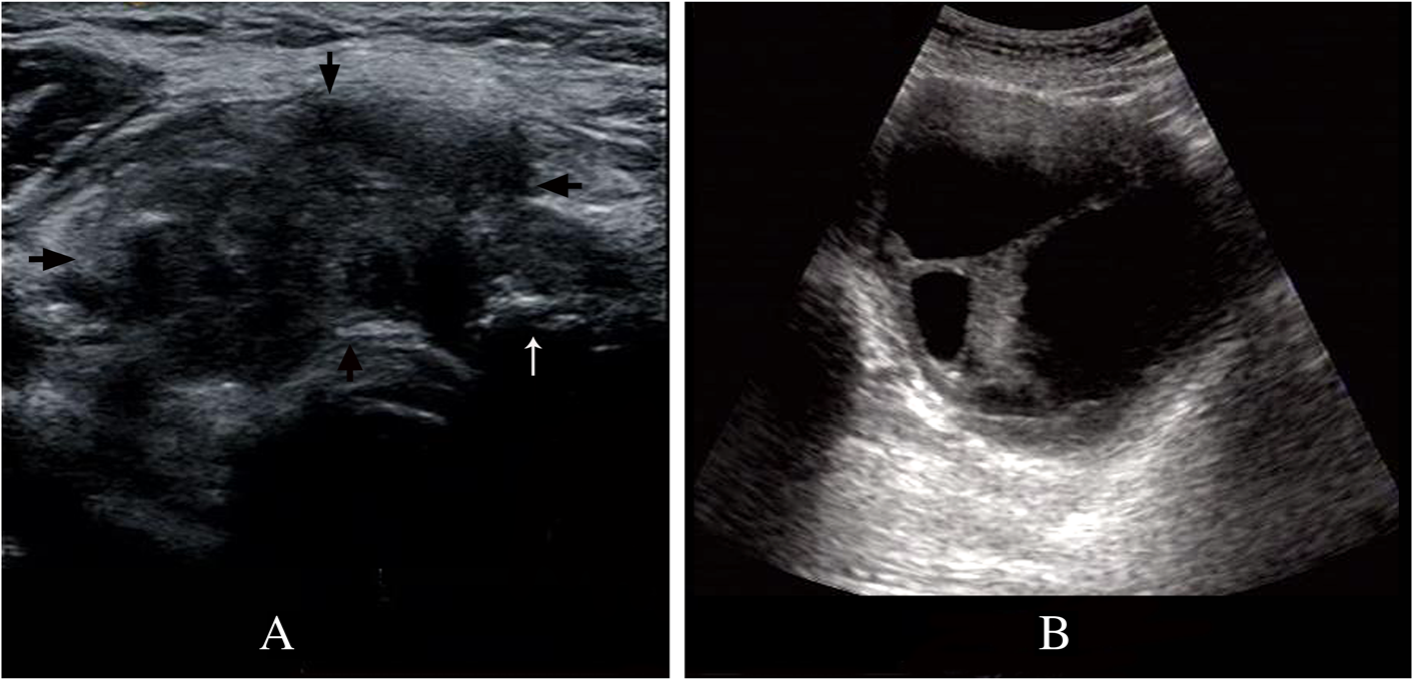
Ultrasound images of the thyroid nodule and the uterine mass. A. A heterogeneous hypoechoic mass was detected in the thyroid. The blurred margins are marked by four arrowheads (→). Coarse calcifications with heavy echoic shadow are highlighted by a white arrow (↑). B. A large mass, containing a sizeable portion of fluid and thick separations, was revealed by ultrasound examination during the pre-operative checkup.
